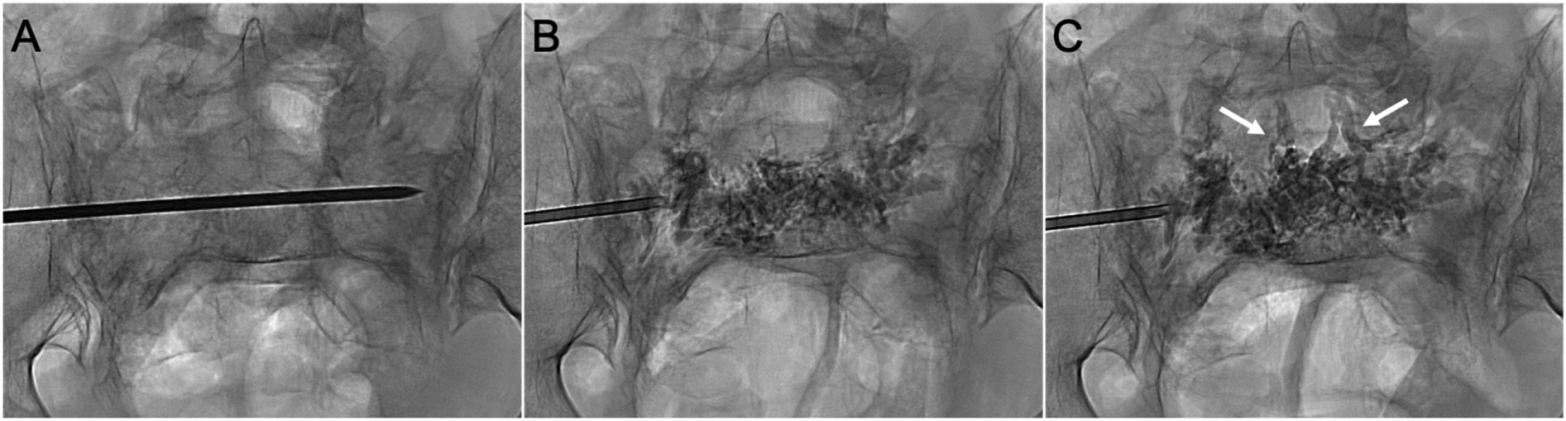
Epidural venous cement extravasation during conventional sacroplasty for bilateral sacral ala insufficiency fractures. (A) PA radiograph shows a transiliac approach at S1. The needle was inserted via the left sacral ala, traversing the sacral body to access the contralateral ala. (B) PA radiograph shows the cement distribution across the sacrum as the needle was withdrawn. (C) Epidural venous plexus extravasation *(arrows)* occurred following additional cement injections in the ipsilateral ala. This resulted from unintended cement propagation along the needle tract, rather than dispersing in the sacral ala around the needle tip.
